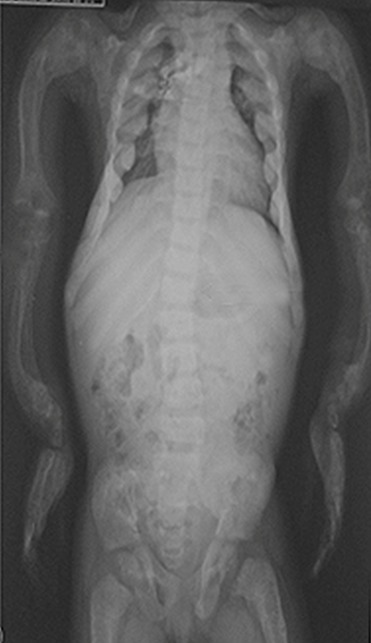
Radiographie thoraco-abdominale de face: transparence excessive des os, minceur de la corticale, déformation en arc des os des deux membres supérieurs, scoliose dorso-lombaire
